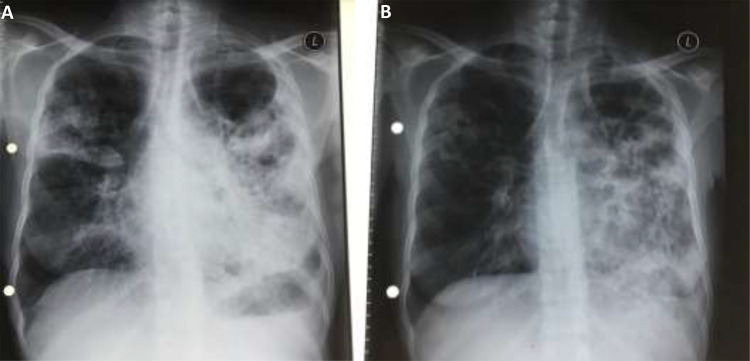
Plain film chest radiographs for patient two - Postero-Anterior view: (A) September 2020, showing extensive bilateral infiltration and cavitation, involving the left lung more extensively than the right, with left upper lobe bullous lesion (B) March 2021, showing fibrosis of the right upper lobe with residual cavities, and fibro-cavitary changes involving of the left lung.
